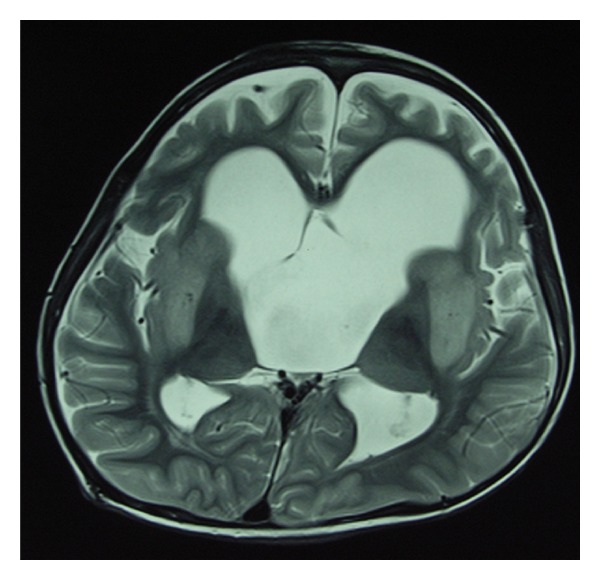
The postoperative MRI image of the patient showed total resection of the cyst lesion, but there existed symmetrical lesions at basal ganglia area (T2 weighted image).
